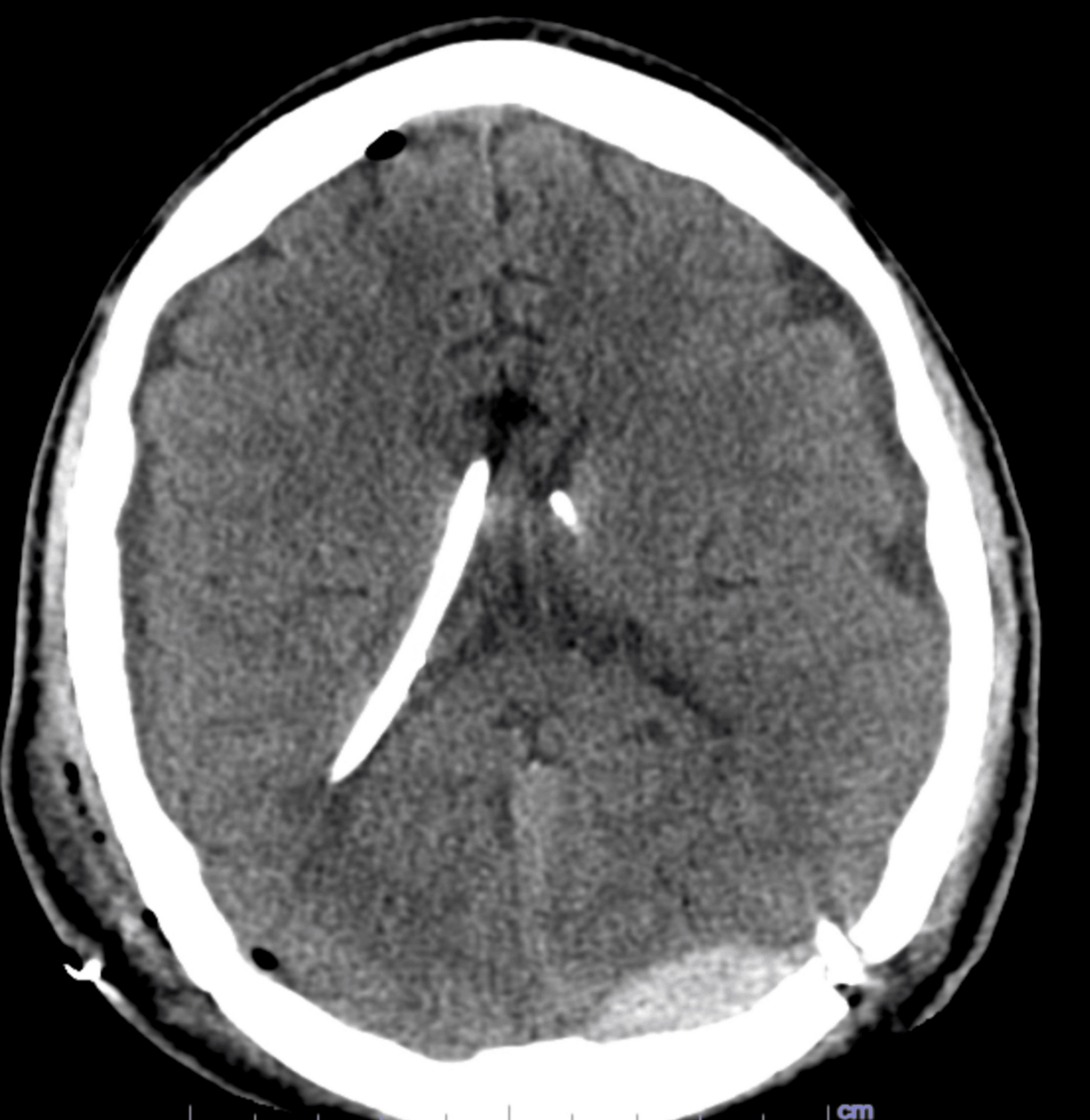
Postoperative axial CT scan showing bilateral ventricular catheter placement with evidence of overdrainage, demonstrated by collapsed lateral ventricles.
